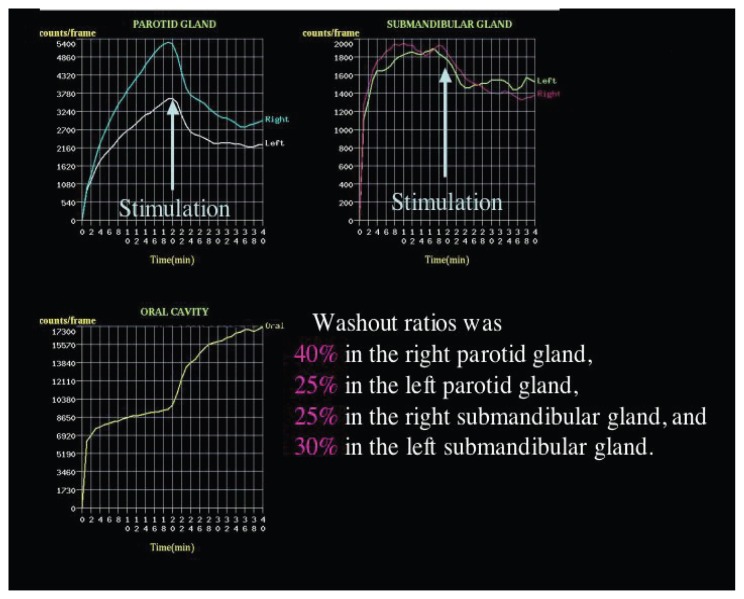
Saliva scintigraphy was performed to evaluate the salivary function before treatment with nizatidine. Washout ratio was decreased in the parotid glands.
